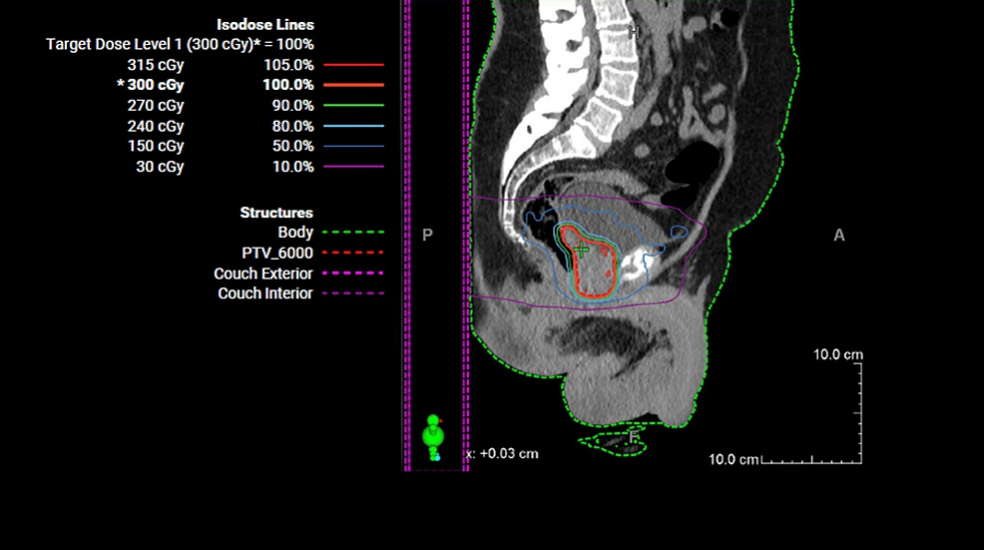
Sagittal CT of the treatment plan for each fraction of radiation to the prostate; 300 cGy in 20 fractions
